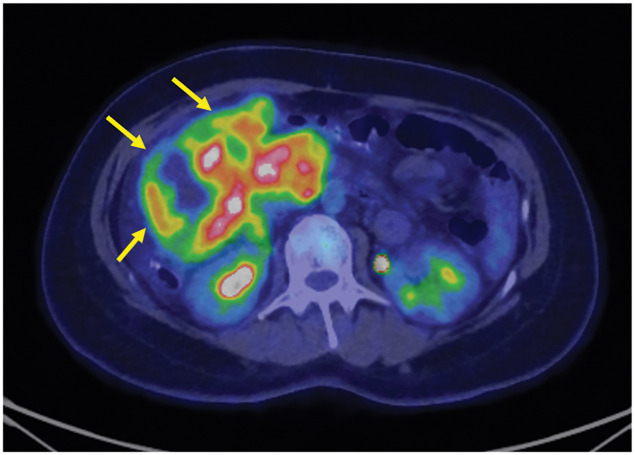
Positron emission tomography-computed tomography showed an irregular accumulation of fluorodeoxyglucose in the tumor (arrows).
